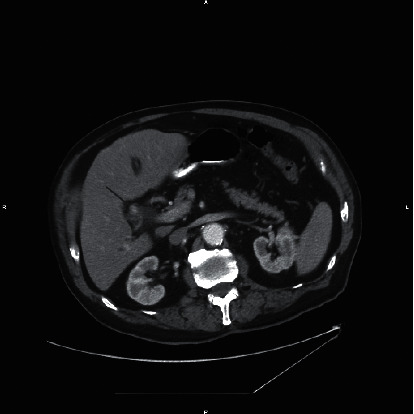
Axial CT of the abdomen with contrast at the level of the junction of the right and left hepatic ducts demonstrates an 11 mm hyperdensity likely representing a calculus (arrow). This finding is confirmed on the coronal image (arrow) in Figure 2.
